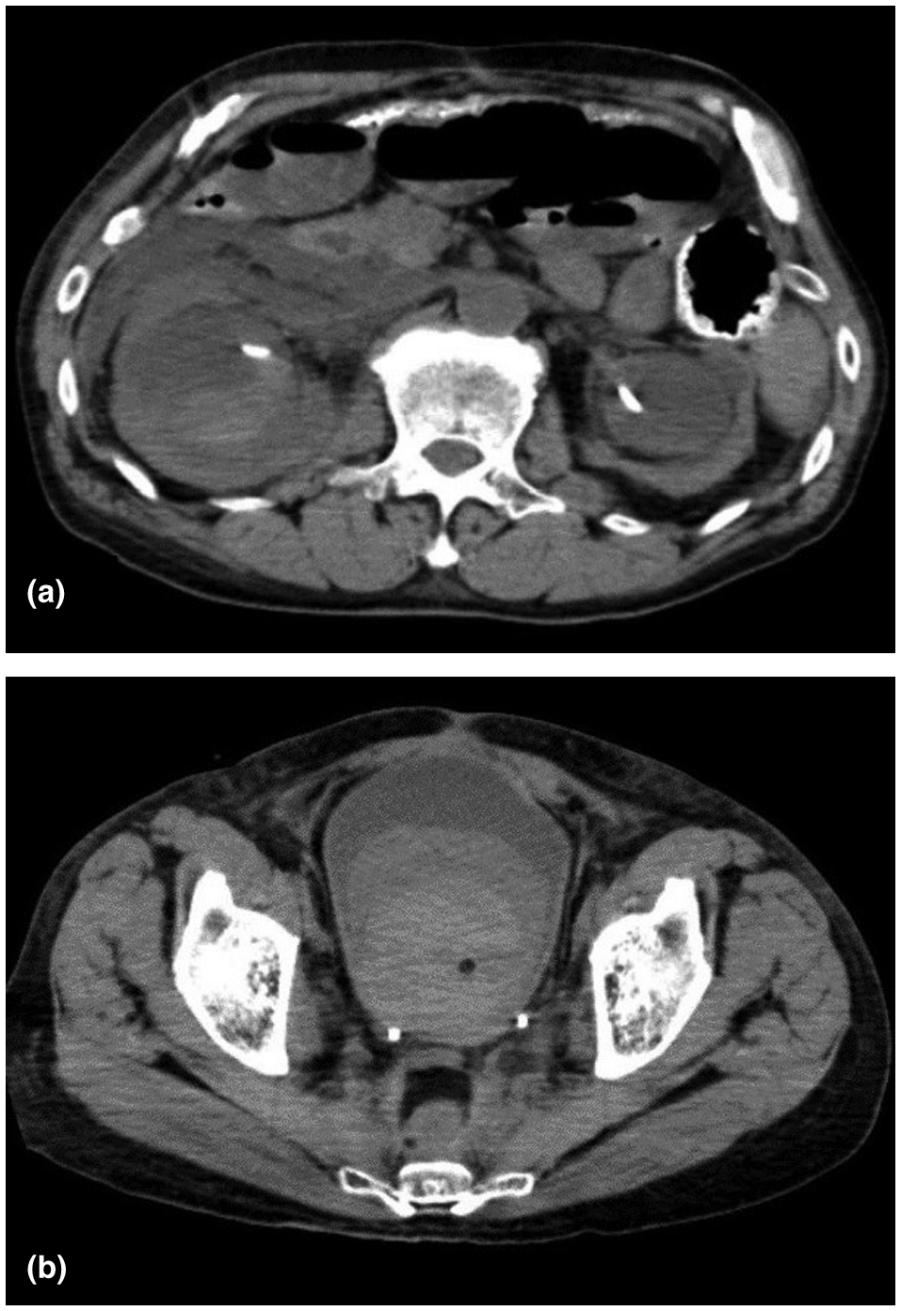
Non‐contrast‐enhanced CT shows hematoma in the right renal pelvis (a) and bladder (b).
